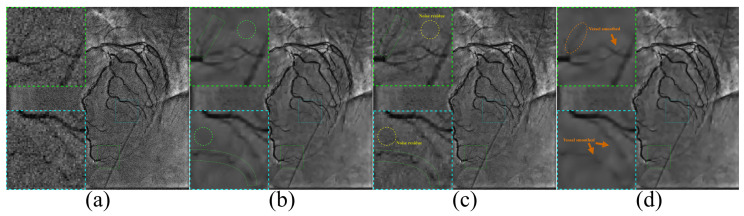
Visual comparison results (a) real-life X-ray angiogram at the 85th frame. (b) Visual result of BM3D with our noise level estimation method (σ=18.6854). (c,d) Visual results of BM3D respectively with empirical noise levels 14 and 24.
